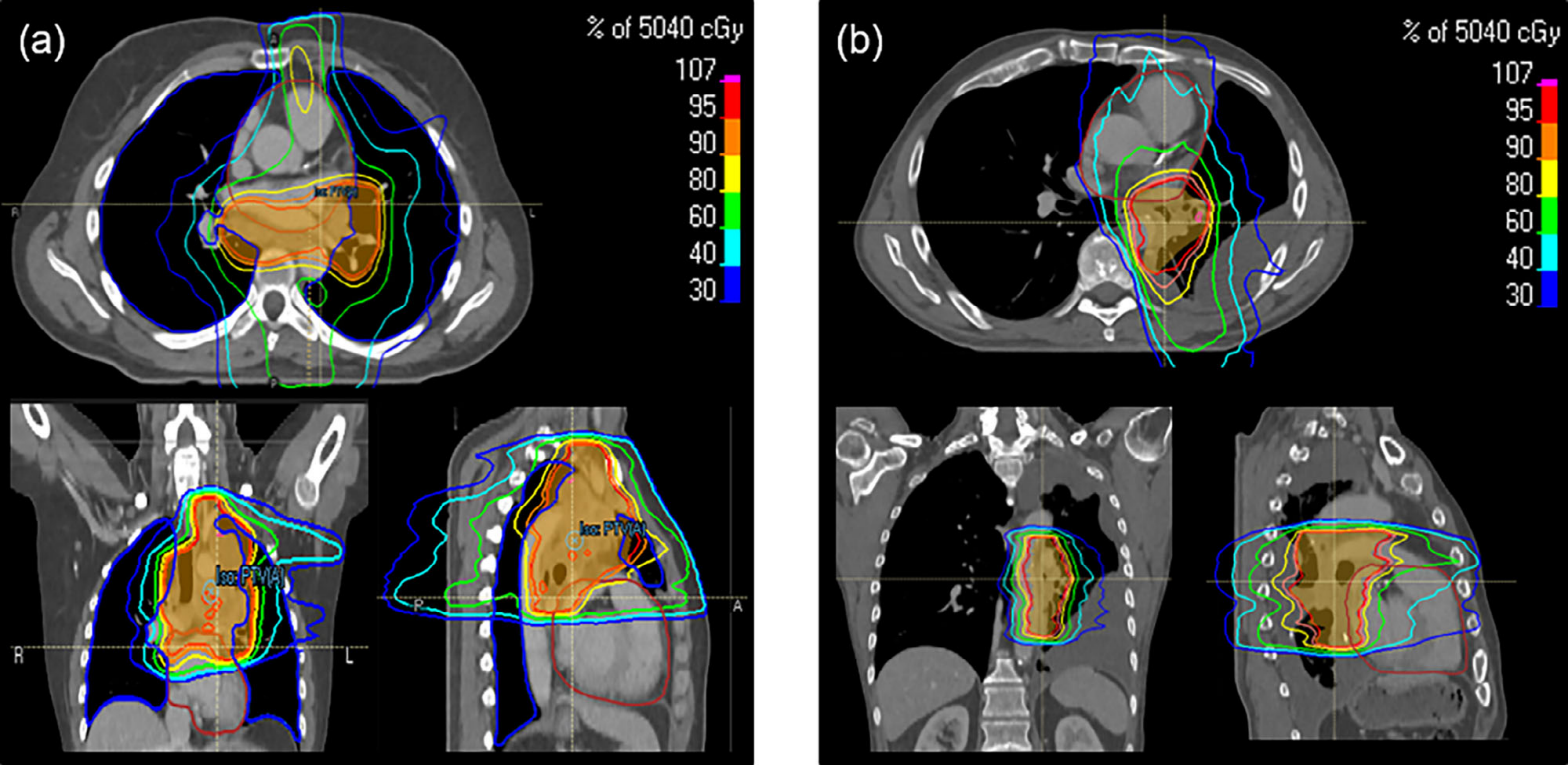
Transverse, coronal, and sagittal plane of CT‐images of two patients with the same heart OVH. The displayed dose distribution and the delivered dose in the heart indicate a dependency on the cranio‐caudal overlap between the heart and the PTV. OVH, overlap volume histogram; PTV, planning target volume.
